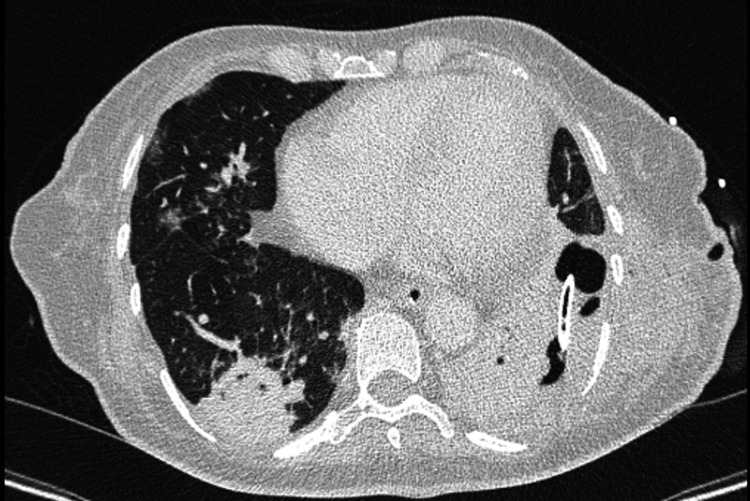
Reassessment thoracic computed tomography Atelectasis with consolidation of the left lower lobe and consolidation involving part of the right lower lobe.
